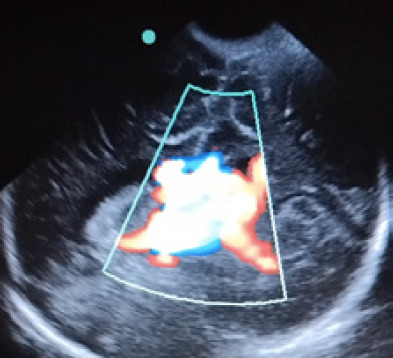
Cranium ultrasound showing dilated intracranial vessels with increased flow.
